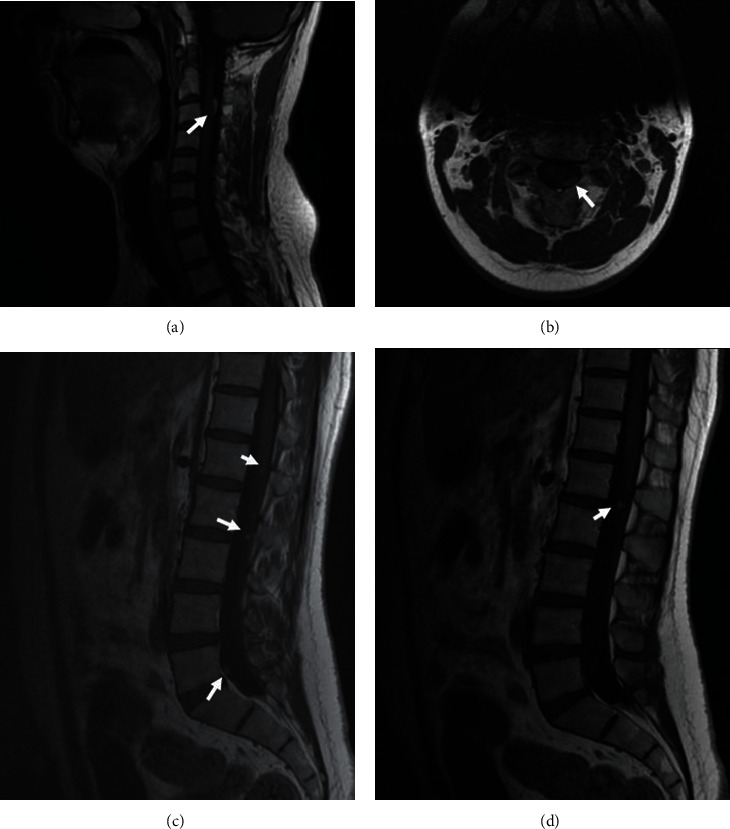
Multisequential multiplanar magnetic resonance images of the spinal cord without contrast. Sagittal (a) and axial (b) T1-weighted MR images reveal an intradural extramedullar mass having a homogeneous high signal intensity, which is consistent with the T1-shortening effect of melanin located at the level of C3 (arrow). Sagittal T1-weighted MR images (c, d) reveal multiple intradural extramedullar small nodules with high signal T1 intensity (arrow) in the cauda equina.
